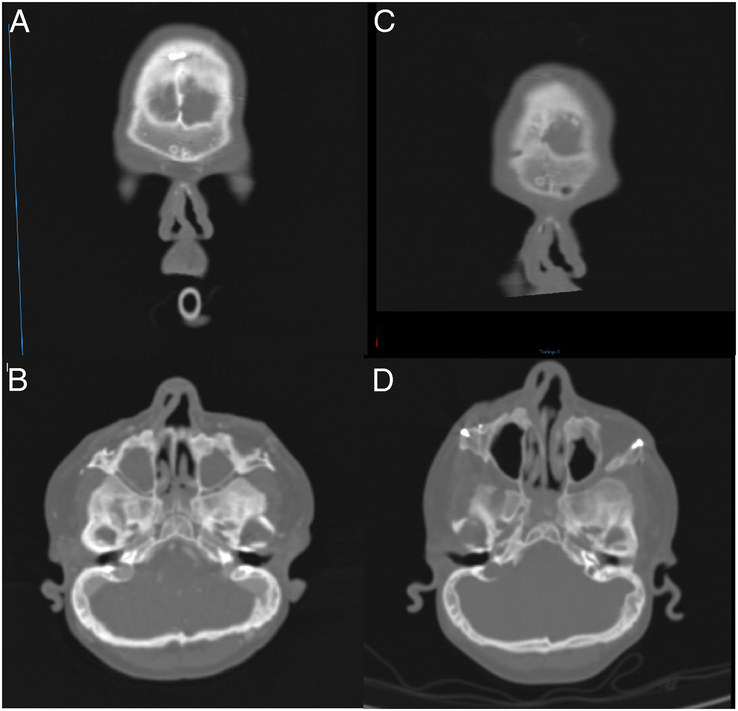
A-D Patient underwent MB with internal distractors at the age of 12. Clinically, she had no deviation of the nose preoperatively, and the nasal position was unchanged in the short-term postoperative photograph. However, both the preoperative and short-term postoperative CT scans of the head showed a pre-existing deviation and a worsening of the deviation, respectively: A. preoperative frontal view showing a C-shaped septal deviation anterior to the ANS, B. preoperative axial view showing a straight external nose and bony septum, but deviated cartilaginous septum, C. 9 moths postoperative frontal view showing a worsened C-shaped septal deviation anterior to the ANS, D. 9 moths postoperative axial view showing a similar image to the preoperative situation.
